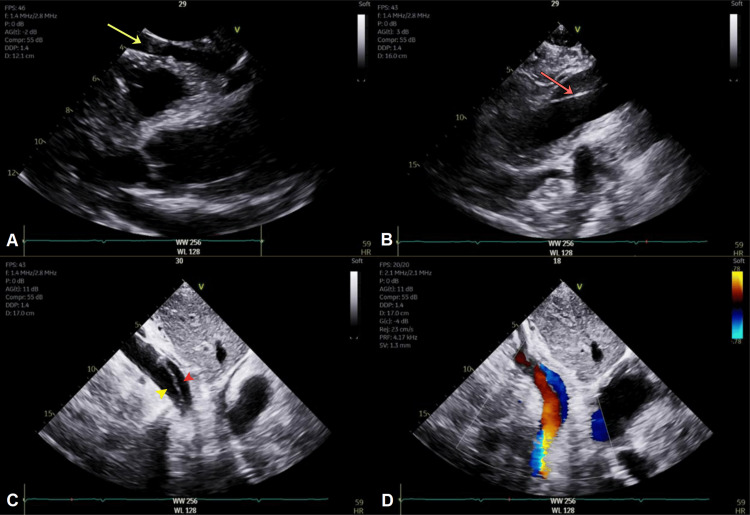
Transthoracic echocardiography demonstrating extensive Stanford type A aortic dissection (A) Zoomed-in parasternal long-axis view shows a normal-sized aortic root with moderate circumferential pericardial effusion (yellow arrow). (B) More superiorly, a mobile intimal flap (red arrow) is visualized within the dilated ascending aorta with a maximum diameter of 4.7 cm. (C) Abdominal aortic view demonstrates separation of the lumen into distinct true (yellow arrowhead) and false (red arrowhead) channels. (D) Color Doppler imaging reveals differential flow between the two lumens, further supporting the diagnosis. Left ventricular systolic function was preserved at 60-65%, with mild aortic regurgitation (not shown)
